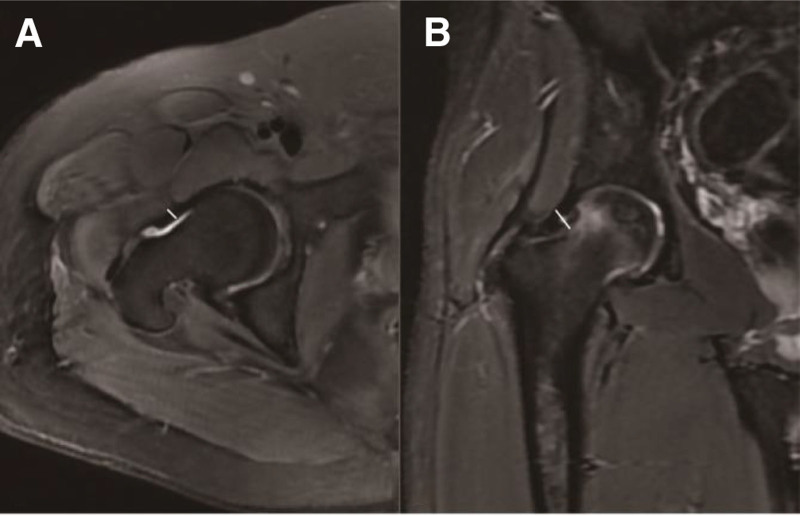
(A) Measurement of the thickness of the anterior capsule at 3:00 on an oblique axial MR image; (B) measurement of the thickness of the anterior capsule at 12:00 on an oblique coronal MR image. MR = magnetic resonance.
